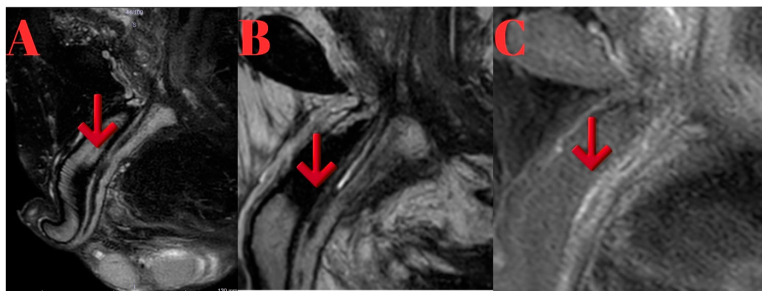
(A,B): T2-weighted sagittal images demonstrating complete obliteration at the distal bulbar urethra (arrows). (C): Post-contrast fat-suppressed T1-weighted sagittal image showing a narrowed lumen with surrounding inflammatory and fibrotic changes (arrow).
